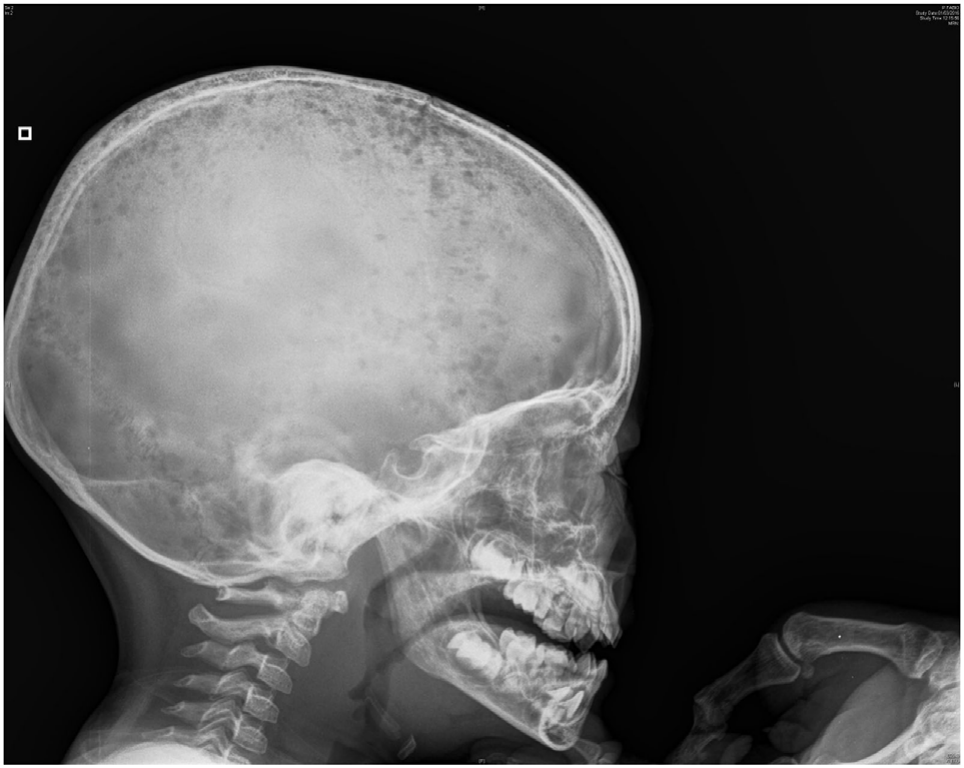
Skull radiograph—multiple lytic areas.
